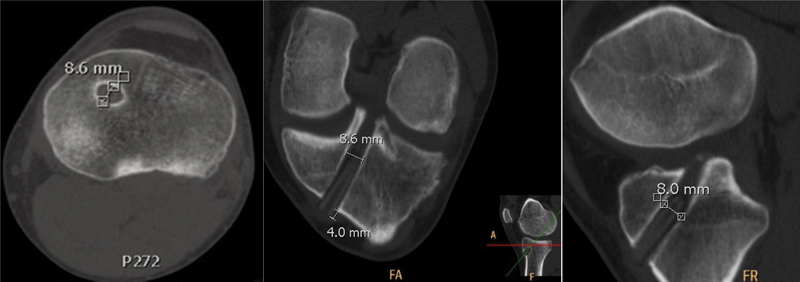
Two-dimensional (2D) computed tomography (CT) measuring method.
